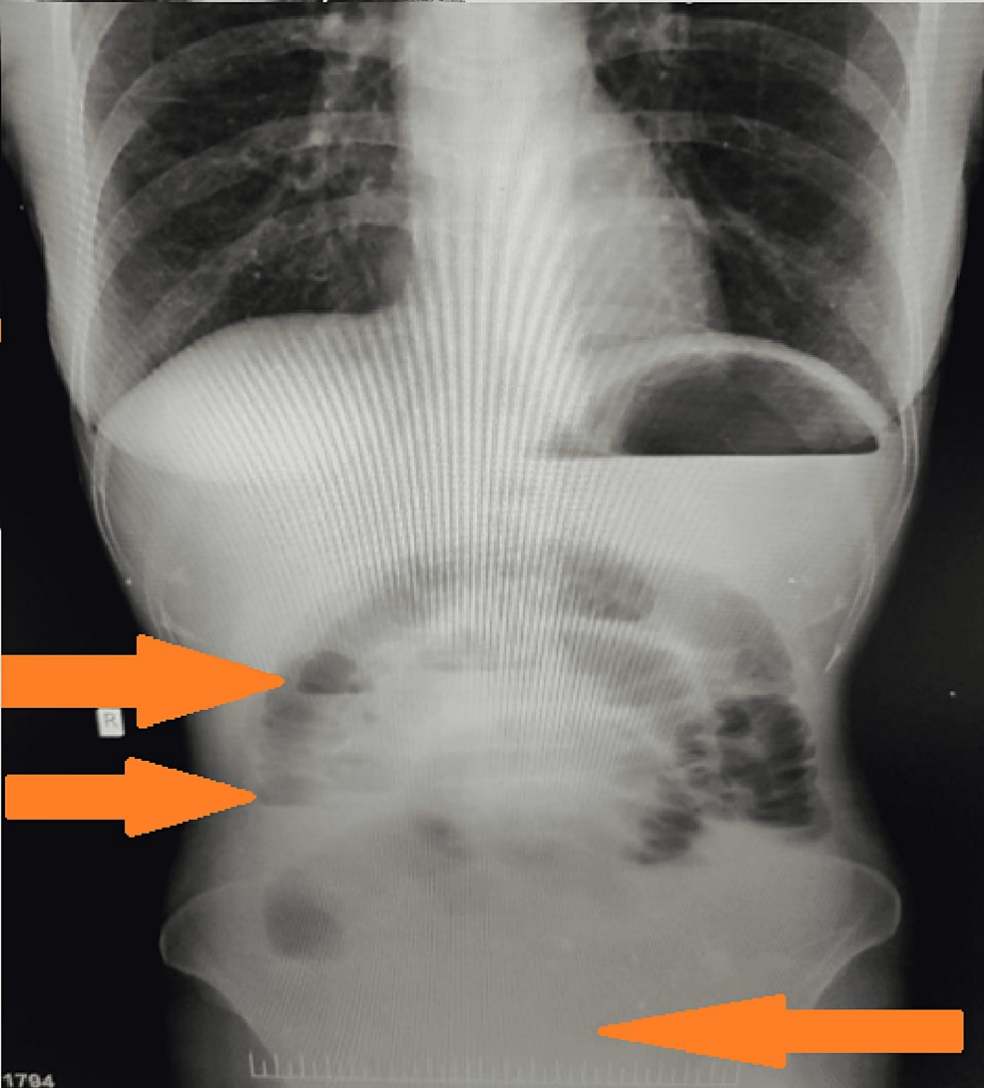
X-ray abdomen (AP erect) showing features of small bowel obstruction (loss of gas in the pelvis and multiple air-fluid levels) (Hazy, as obtained from the emergency department) AP: anteroposterior
